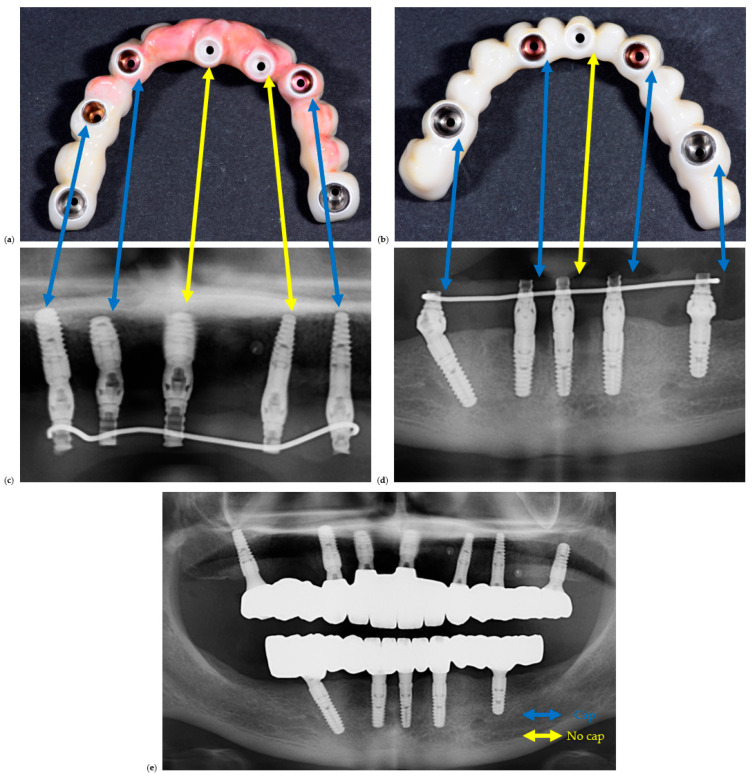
Clinical and radiographic comparison of screw-retained full-arch restorations fabricated with and without prosthetic caps. (a) Clinical photo of a maxillary full-arch screw-retained restoration, (b) clinical photo of a mandible full-arch screw-retained restoration, (c) section of an OPG corresponding to (a), (d) section of an OPG corresponding to (b). Blue lines are between implant sites with caps, while yellow lines are between implant sites with caps. (e) is the OPG of the final delivered restorations.
